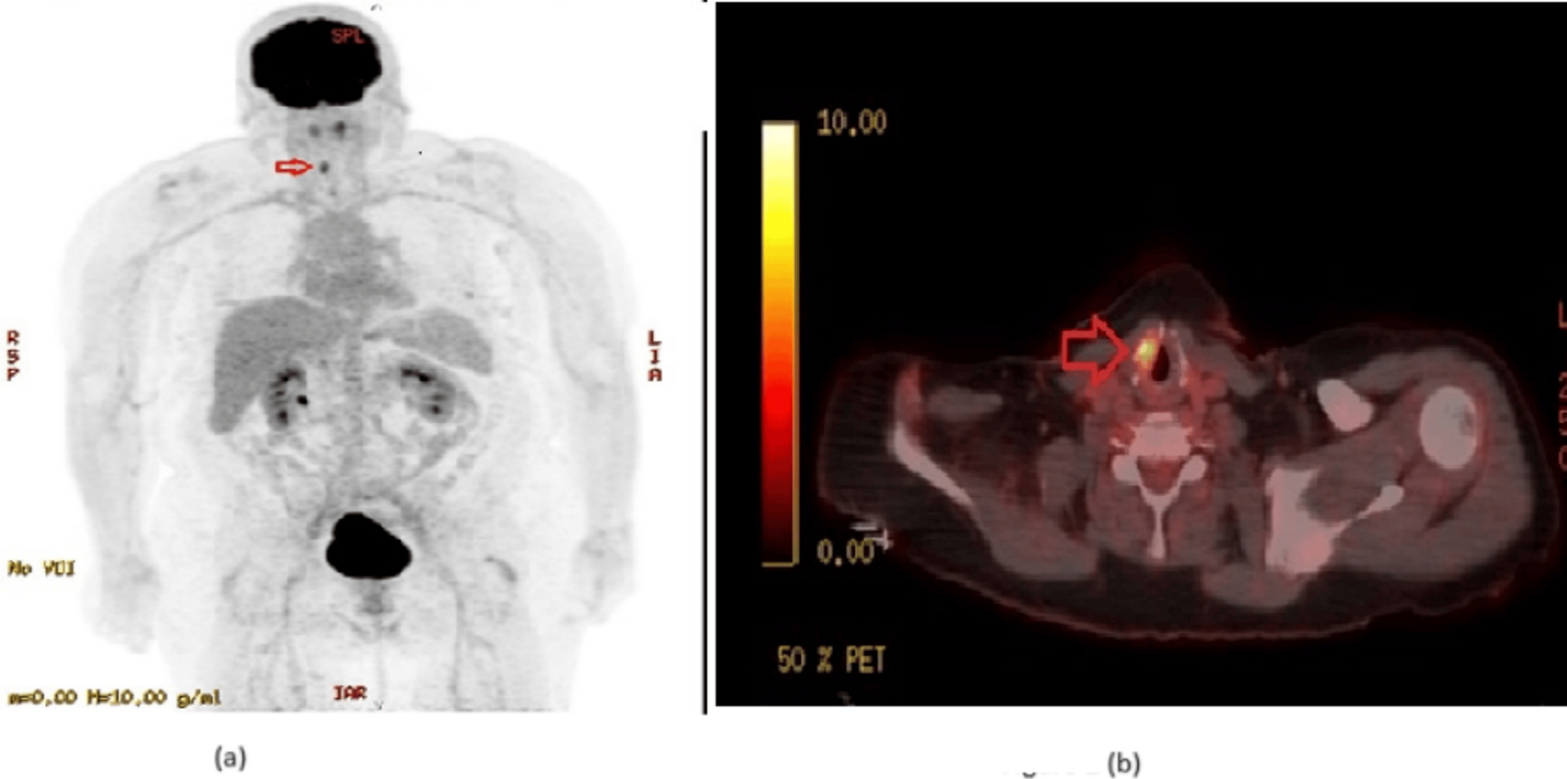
Fluorodeoxyglucose (FDG) avid right-sided vocal cord PET scan showing the reactive right-sided laryngeal node in (a) coronal plane and (b) transverse plane
